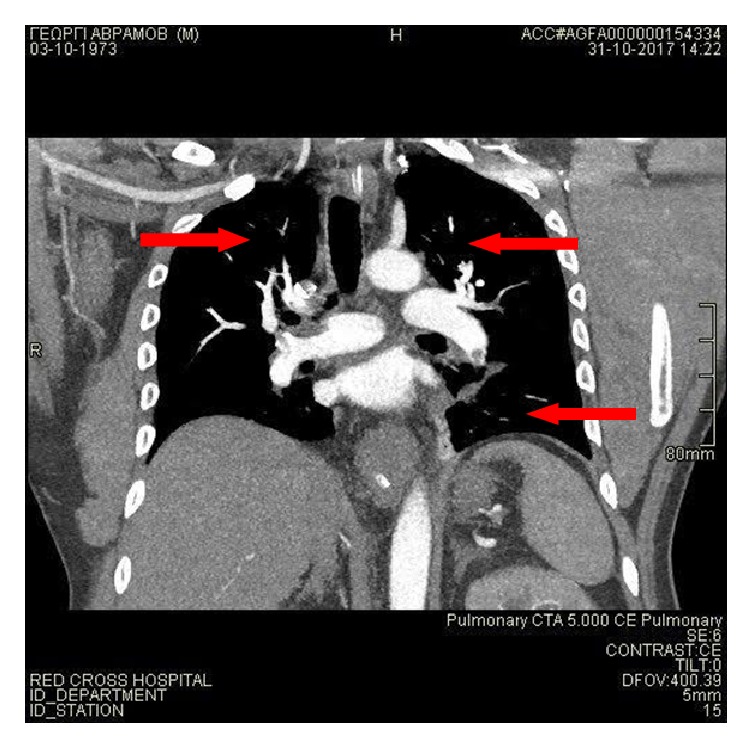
Chest CT angiography scan, depicting filling defects in subsegmental branches of the right pulmonary lobes, in segmental branch of the left lower pulmonary lobe, and in subsegmental branches of the left upper pulmonary lobe.
